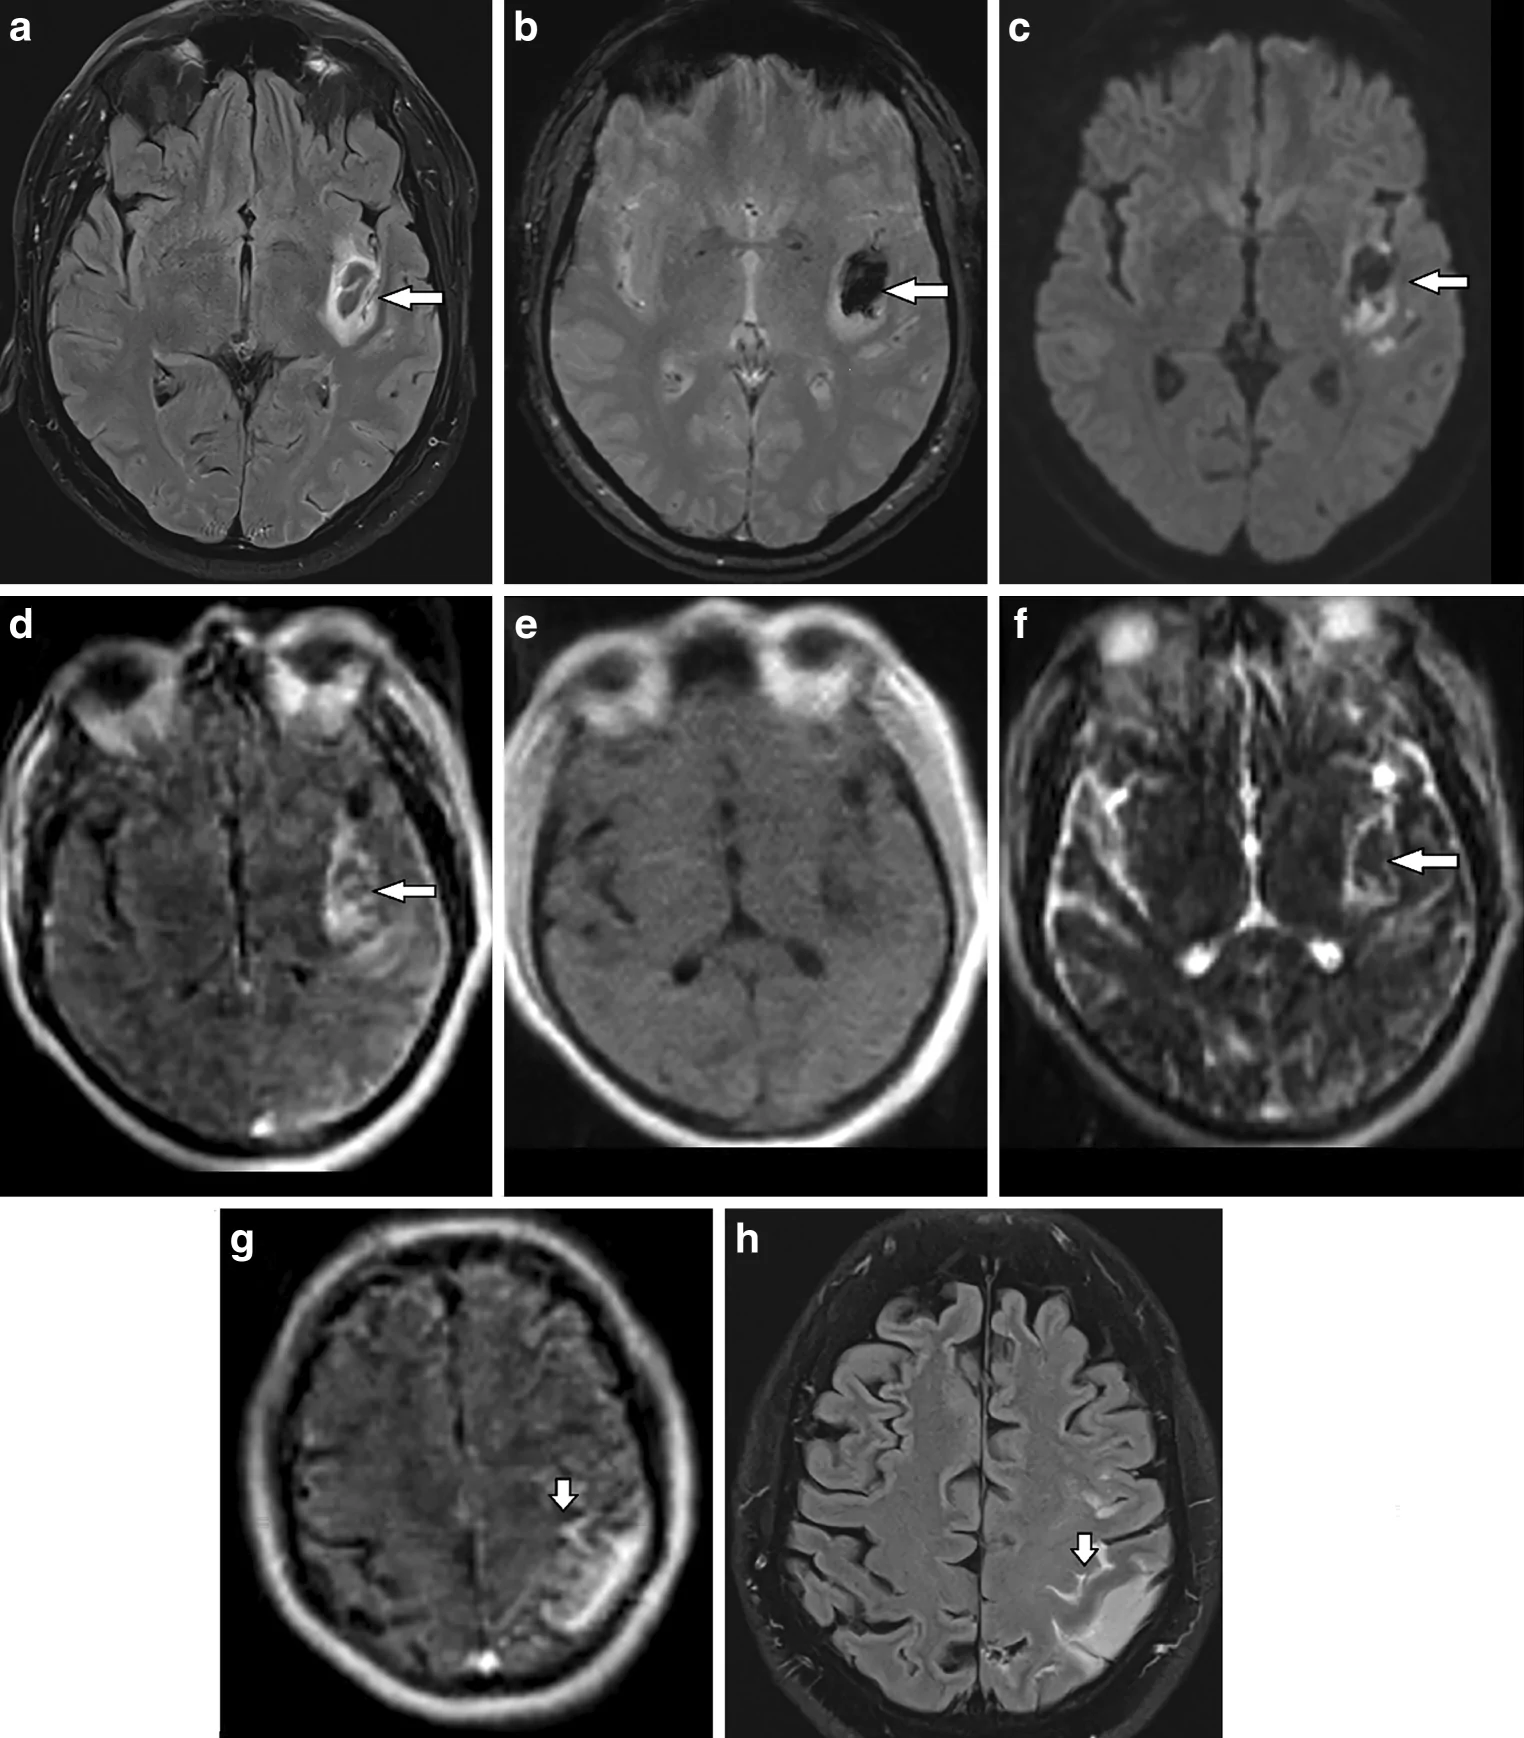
A 59-year-old patient with left in the middle cerebral artery territory ischemia due to M2 occlusion showing an early subacute infarction involving the left insula with hemorrhagic transformation and associated subarachnoid hemorrhage. ULF-pMRI demonstrated a large left insular parenchymal hemorrhage without significant mass effect (HBC 1c; arrows in a–c: Standard MRI, d–f: ULF-pMRI) and widespread subarachnoid hemorrhage (HBC3c) extending to the right central sulcus, visible as FLAIR hyperintensities (arrows in g and h). Standard MRI was acquired one day after ULF-pMRI
